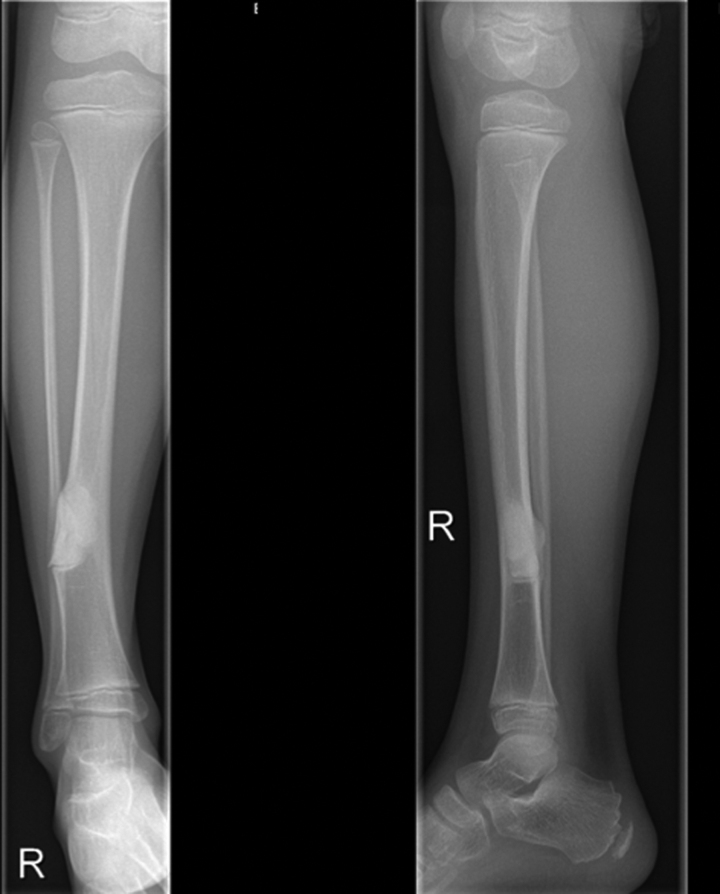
Radiographic image at the age of 9 showing the cement plombage after marginal resection of the tumor.
